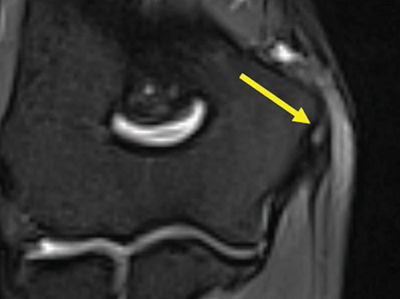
Coronal fat-saturated T2-weighted image through the medial flexor origin with an intratendinous T2-hyperintense signal, score 2.
